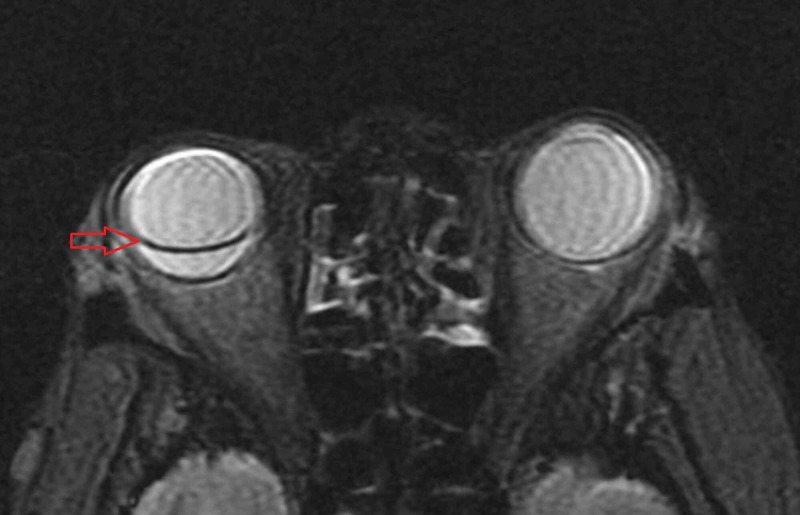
MRI-orbit Right eye retinal detachment (red arrow)
